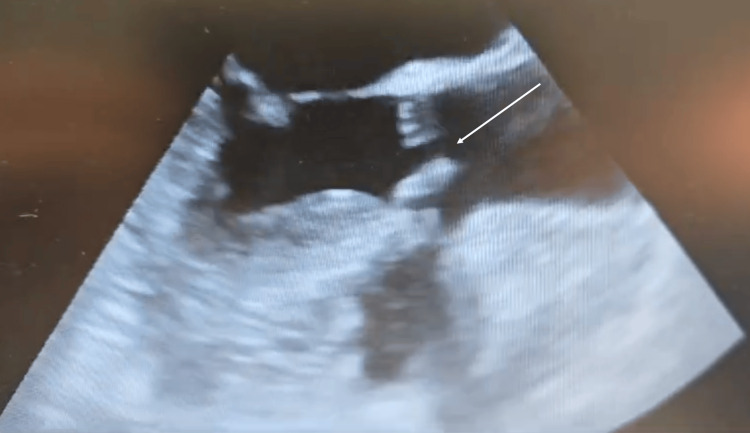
TTE parasternal long-axis view revealing severe aortic stenosis TTE: Transthoracic echocardiography
